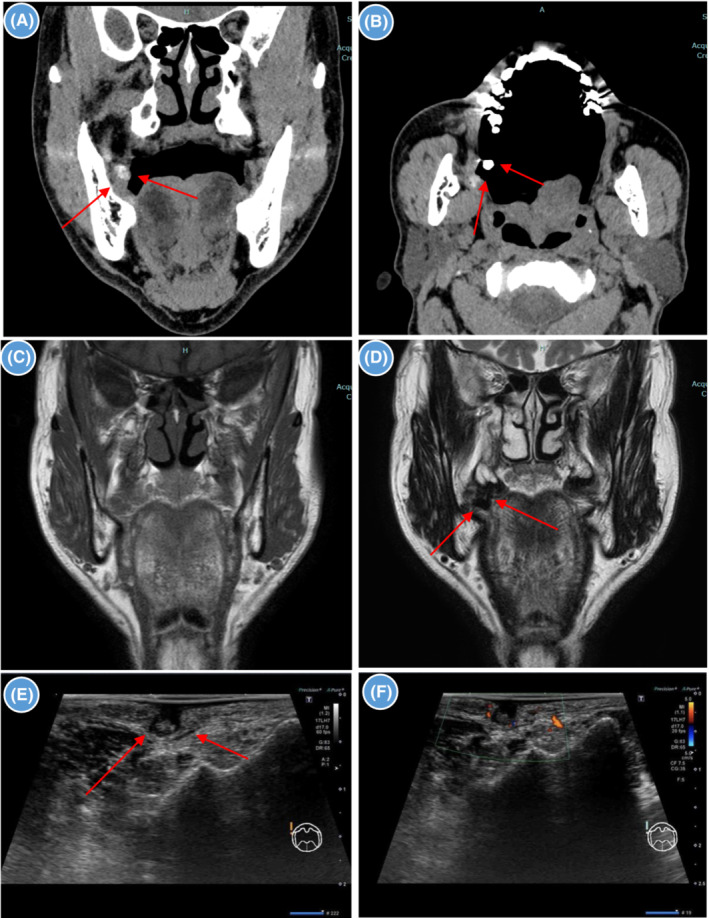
Radiological images. (A, B): Contrast‐enhanced computed tomography images showing an area of contrast under the buccal mucosa (arrowhead). (C, D): Magnetic resonance images showing an oval nodule with a poor contrast effect under the buccal mucosa; there is no signal on the T1‐weighted image and low signal on the T2‐weighted image (arrowhead). (E, F): An ultrasound scan showing a hypoechoic mass in the right side of the buccal mucosa. The interior of the mass is isoechoic to hypoechocic (arrowhead). Color Doppler examination did not reveal increased blood flow signal in the mass.
